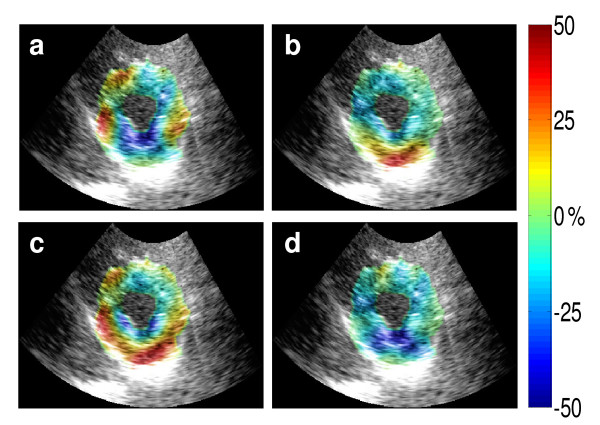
Transthoracic echocardiography and elastography of a healthy human left ventricle. (a), (b), (c), and (d) are the lateral, axial, radial, and circumferential systolic strains from myocardial elastography between end diastole and end systole, respectively. Strains are displayed on a scale of ± 50%. All the images were acquired approximately at the papillary muscle level and shown at end systole. Contributed by Elisa Konofagou (Columbia University, New York).
